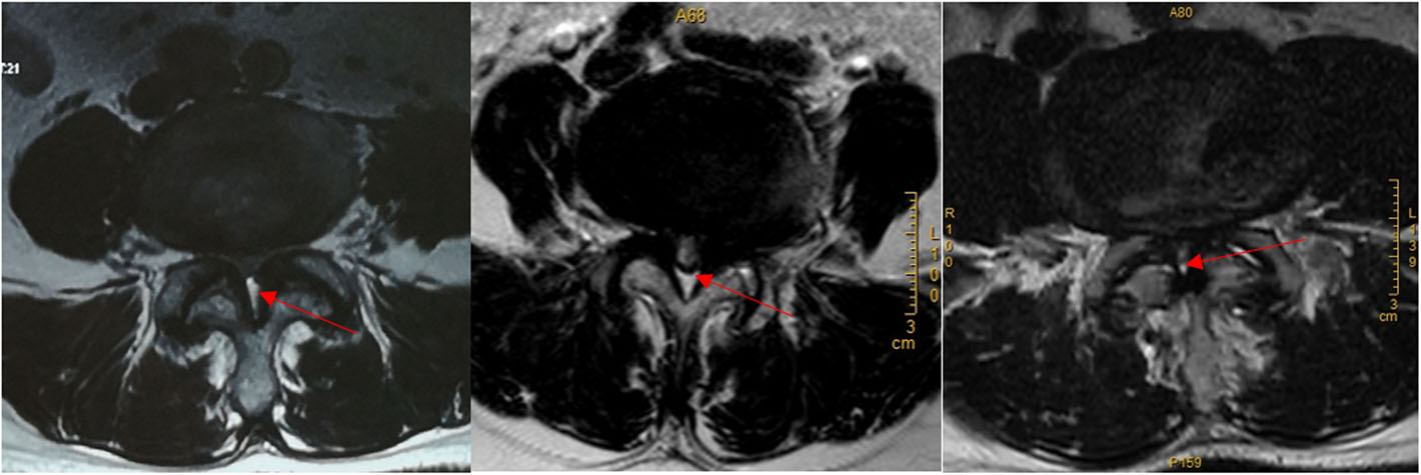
Even for severe lumbar spinal stenosis, adipose tissue can still be seen on the dorsal side of the dural sac in axial MRI images (Red arrow: adipose tissue). The pathogenic factors of stenosis mainly come from bilateral and ventral compression
